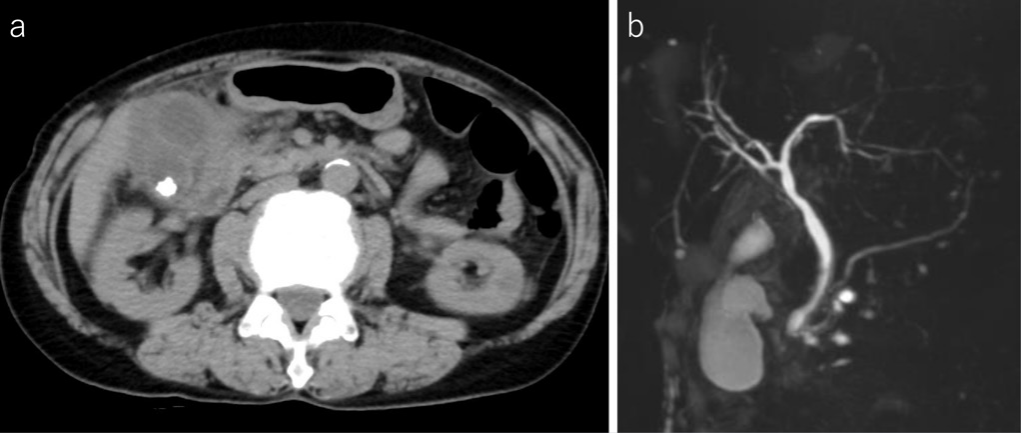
Abdominal plain CT (a) and MRCP (b) on initial admission. Plain CT (a) showed multiple stones, swelling, and wall thickening of the gallbladder. The anteroposterior view of MRCP (b) does not clearly show a biliary circuit and cystic duct (arrow). MRCP, magnetic resonance cholangiopancreatography.
